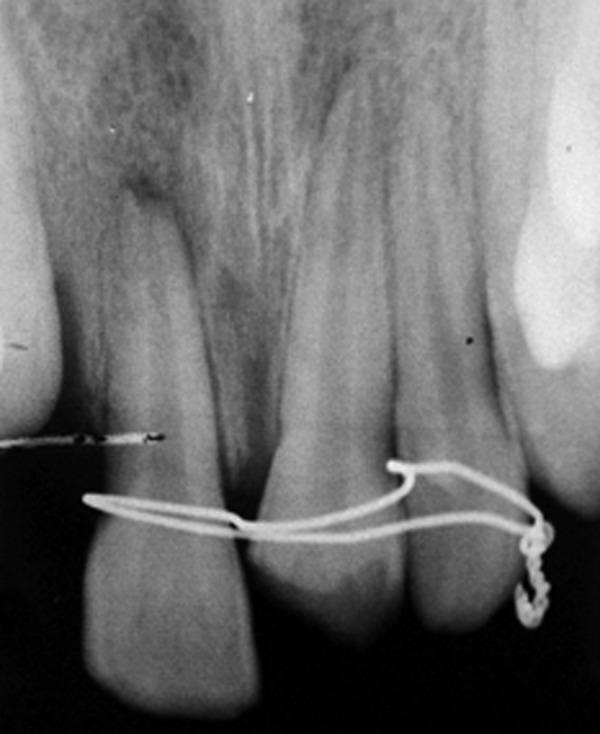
Preoperative intraoral periapical radiograph (case 3)
